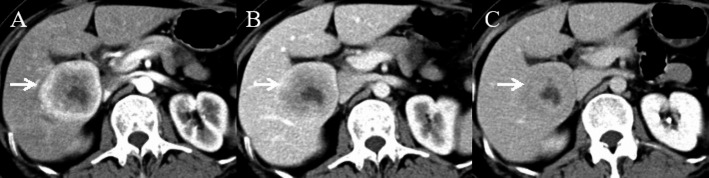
Contrast-enhanced CT of the lesion. A Arterial phase ring-like enhancement resembling massive HCC. B Portal venous phase with reduced enhancement. C Delayed phase with central fill-in and non-enhancing areas, reinforcing suspicion of primary liver cancer
